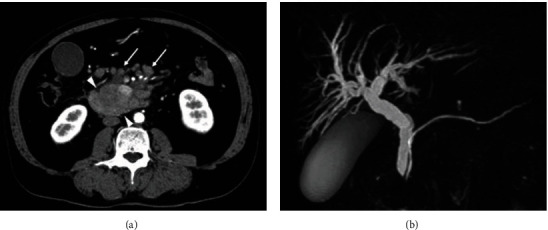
(a) Computed tomography image (pancreatic parenchymal phase). A slightly enhanced, relatively homogeneous tumor can be seen in the pancreas head (arrowheads). Multiple enlarged peripancreatic and mesothelial lymph nodes (arrows) are visible. (b) Magnetic resonance cholangiopancreatography image showing bile duct dilatation.
